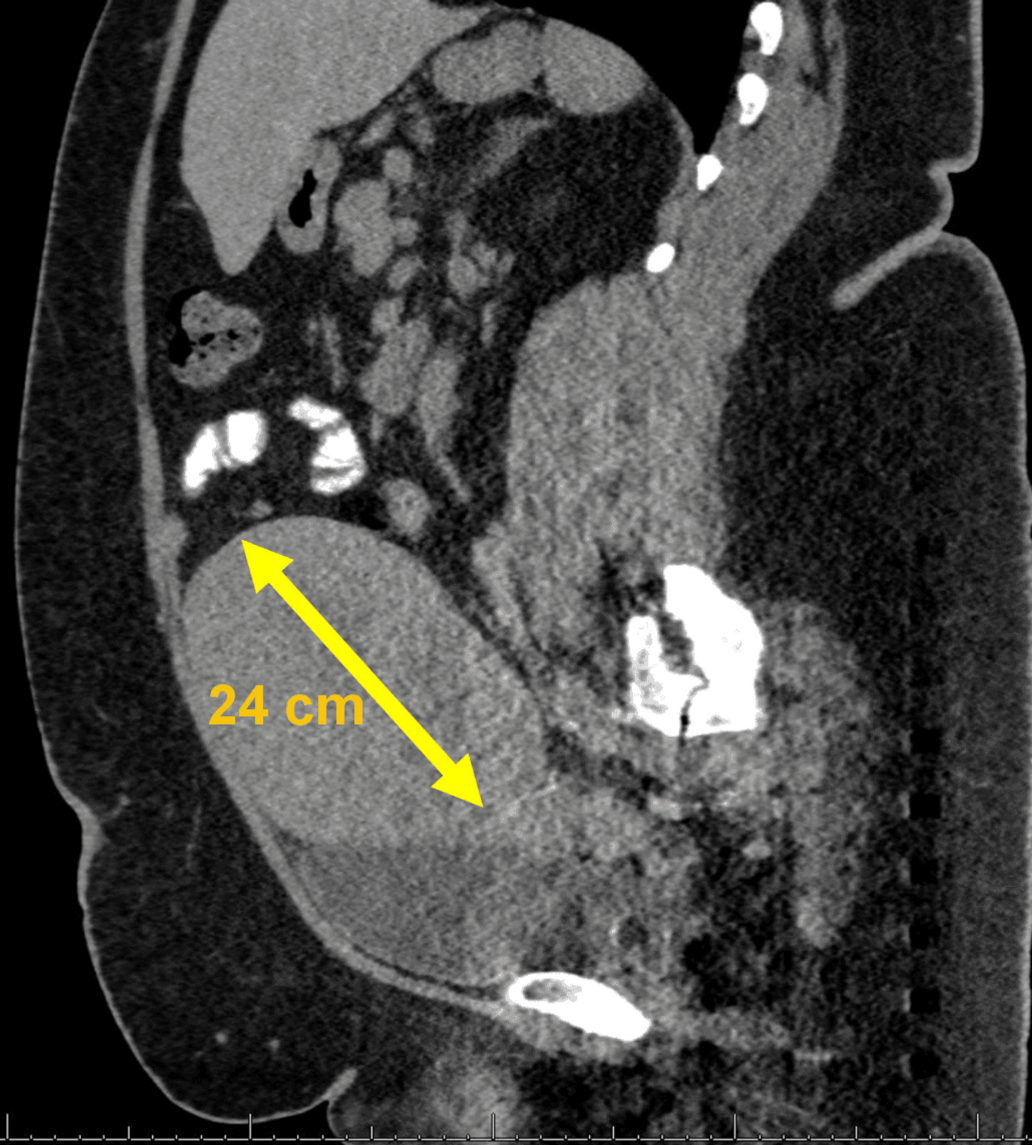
Sagittal view of a CT of the abdomen and pelvis The CT scan image reveals a uterus measuring approximately 24 cm in length.
